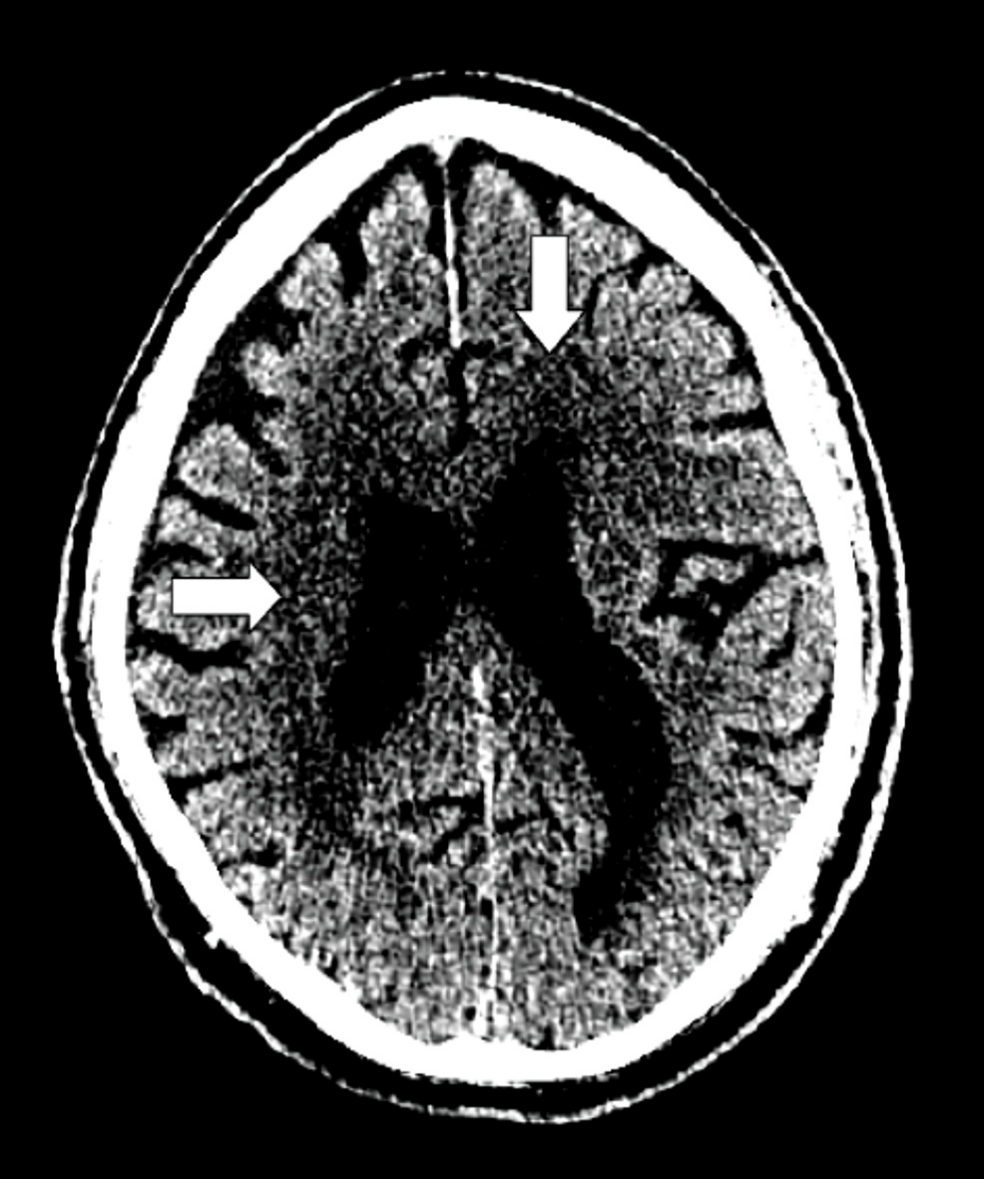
Thin standard view of the CT brain without contrast demonstrates diffuse periventricular and subcortical white matter hypodensities as indicated by the white arrows
